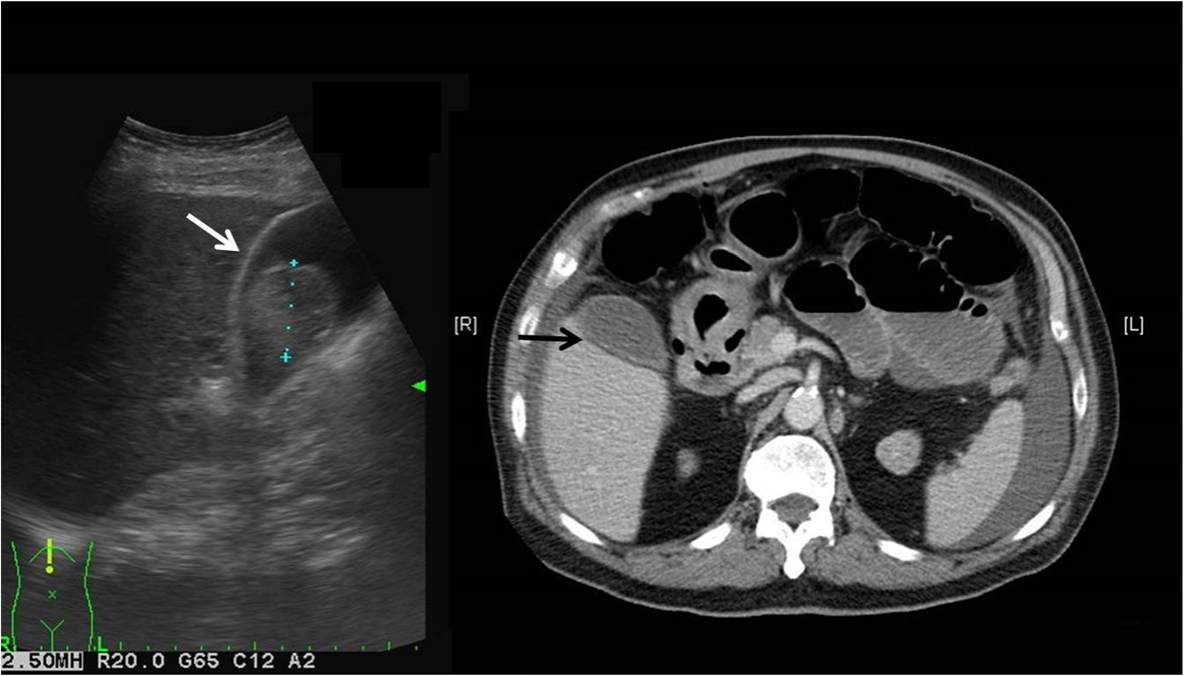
Abdominal ultrasonography revealed distended gall bladder with sludge within it without wall thickening (white arrow); Abdominal computed tomography scan revealed dilated bowel with air-fluid level, much fluid around spleen and a distended gallbladder with modest thickening of the wall (black arrow)
